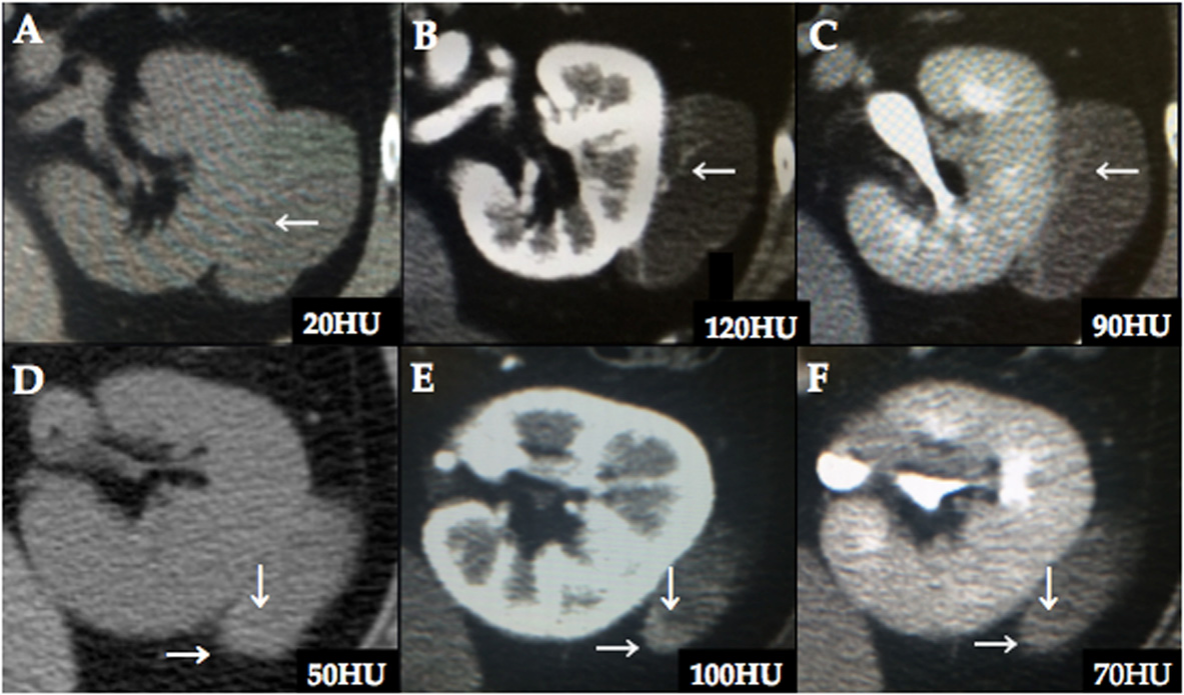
Preoperative computed tomography. The renal mass appears mainly as a hypodense area (a) with a partially hyperdense area (d) on unenhanced computed tomography. The hyperdense area and septa are enhanced in the early phase (b, e) and washed out in the late phase (c, f). White arrows show the area where CT attenuation value is calculated
